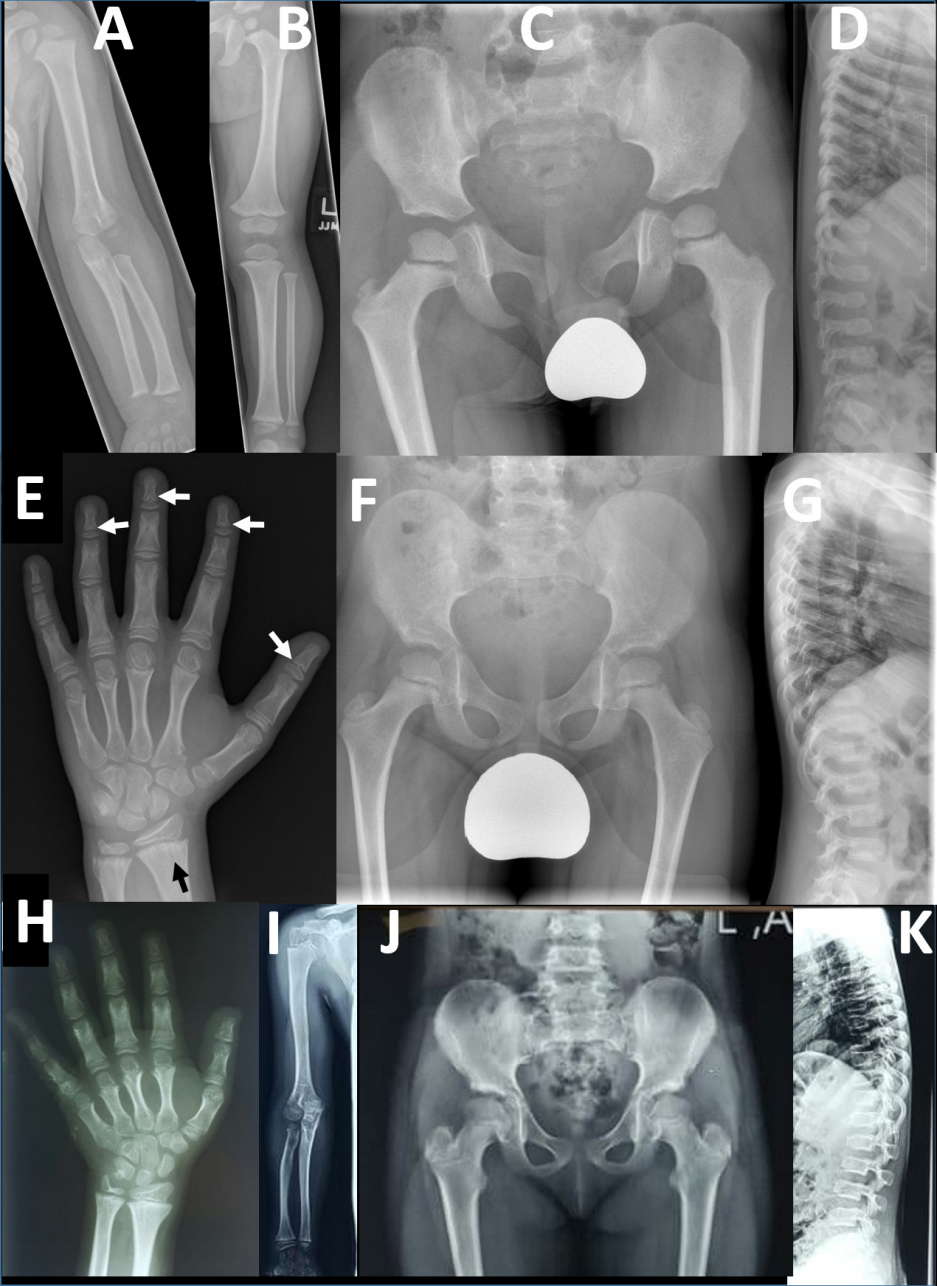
Radiographic findings in two families with *PRKG2* variants: radiographs of left upper limb (A) and lower limb (B) in a 26-month-old boy (F1-IV-7) from family 1. The long bones are stocky in appearance but there is no disproportion within the limbs. (C) Pelvic radiograph at age 4 in same child shows development of long, slender femoral necks. (D) Lateral spinal radiograph at age 4 show generalised mild platyspondyly with small central anterior projections of the vertebral bodies, and hypoplasia of the L2 vertebral body. (E) Left hand radiograph at age 11 in same child shows no brachydactyly; there is mild metaphyseal chondrodysplasia evident in the distal radius and particularly the ulna, with some metaphyseal striations (black arrow); subtle coning of the distal phalangeal metaphyses is evident (white arrows), without associated shortening. Pelvic (F) and lateral spine (G) radiographs in middle affected sibling (F1-IV-6) in family 1 showing similar features of long slender femoral necks and platyspondyly with anterior vertebral body projections. Osteopaenia is also evident; this child also has type 1 osteogenesis imperfecta due to a *de novo* pathogenic variant in *COL1A1*. Additional radiology is available for F1-IV-3 in online supplemental figure 5 which shows similar results to those for F1-IV-7. (H) Left hand radiograph in female child (F2-V-3, aged 10 years) from family 2 showing generalised brachydactyly. (I) Right upper limb radiograph also from F2-V-3 demonstrates mild disproportionate shortening of the radius and ulna relative to the humerus (mesomelic shortening). (J) Pelvic radiograph from F2-V-3 demonstrates mildly elongated femoral necks. (K) Lateral spine radiograph from the same individual demonstrates mild platyspondyly with small anterior vertebral body projections.
